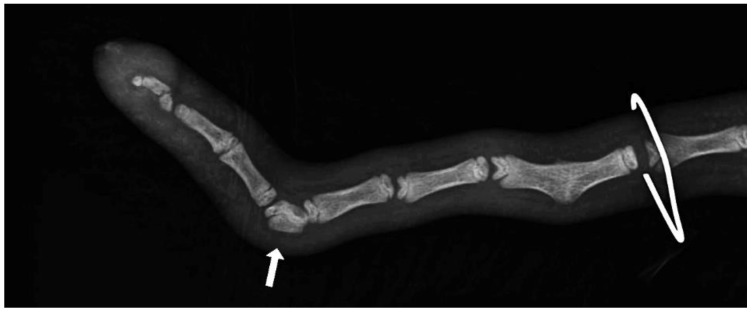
Section of an X-ray of a lamb’s tail with a wedged vertebra.
